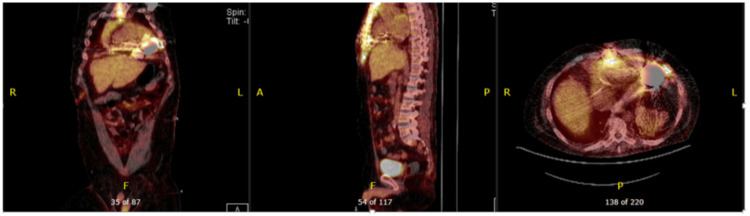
LVAD (HeartMate 3) with Candida albicans infection. 18F-FDG PET-CT showed FDG activity in the pericardium, mediastinum, surrounding LVAD pump, driveline, and sternum consistent with deep and superficial LVAD infection. Reprinted under the terms of the Creative Commons Attribution 4.0 International License from Aguilera et al., Front. Cardiovasc. Med., 2021 [62]. LVAD: left ventricular assist device
